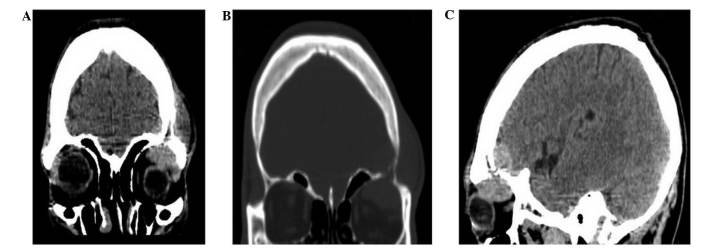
Computed tomography imaging of the patient, including: (A) A coronal plane image demonstrating intracranial and extracranial masses in the left orbit region under the scalp; (B) a coronal plane image indicating lytic changes in the skull bone; and (C) a sagittal plane image showing a cranio-orbital mass.
